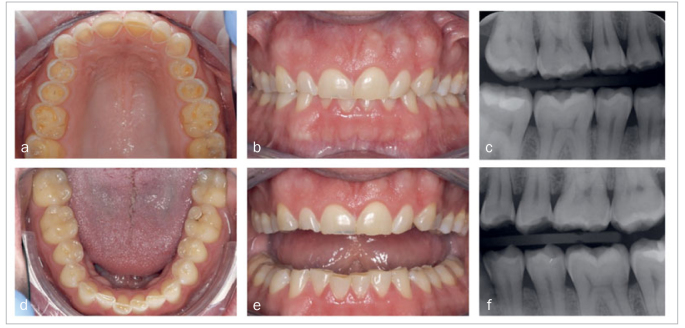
Intraoral photographs and bitewing radiographs at baseline.
